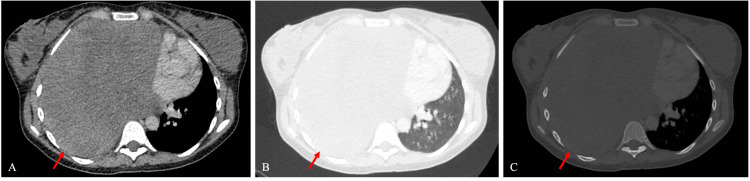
A contrast-enhanced computed tomography (CT) scan showing a large, low-density mass in the right hemithorax with moderate mediastinal shift towards the left (red arrow). There was no obvious destruction of adjacent bones. Panel A shows the mediastinal window, demonstrating the chest wall and pleura. Panel B shows the lung window, demonstrating the lung parenchyma in detail, including the pulmonary vasculature. Panel C shows the bone window.
